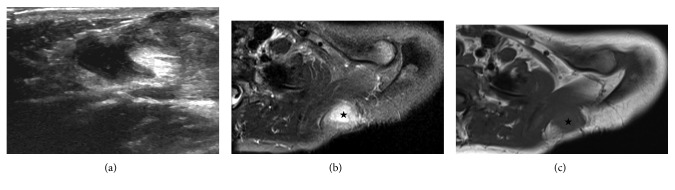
Radiologic images of the soft tissue lesion (star) from ultrasonography (a) and axial MRI ((b) *T1 weighted* and (c) *T1 fat suppressed*).
